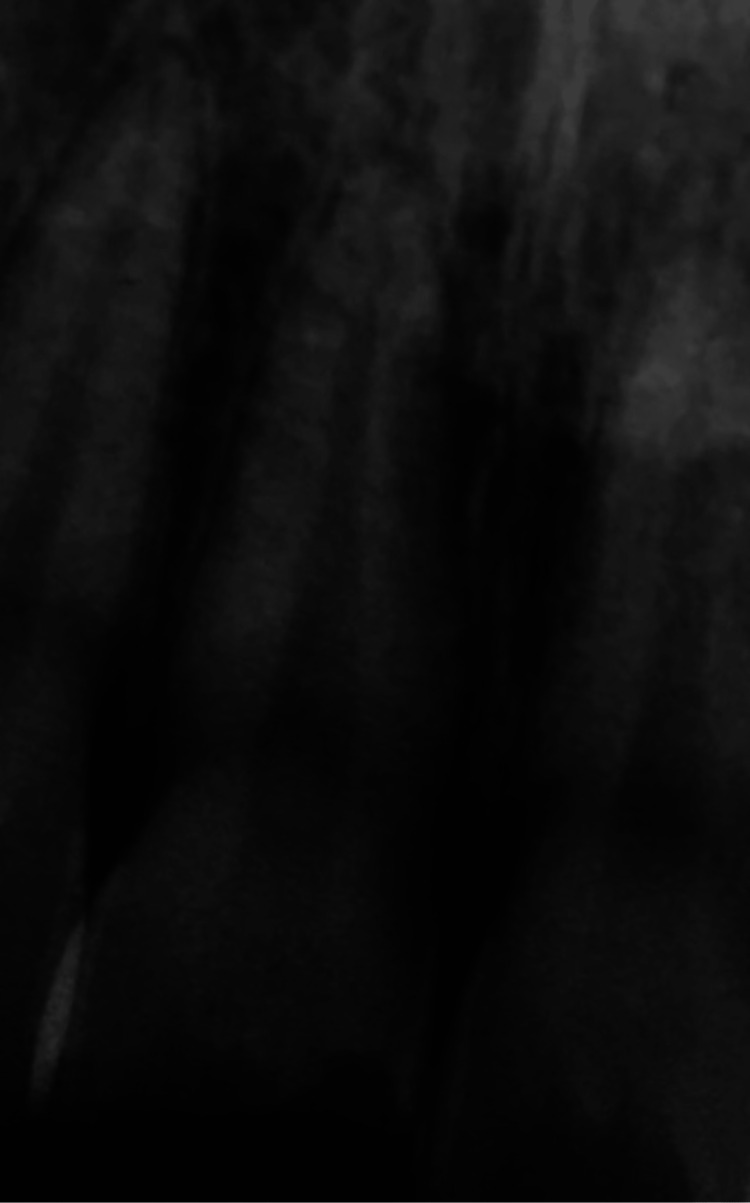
Radiograph of fractured 11 to rule out a root fracture
